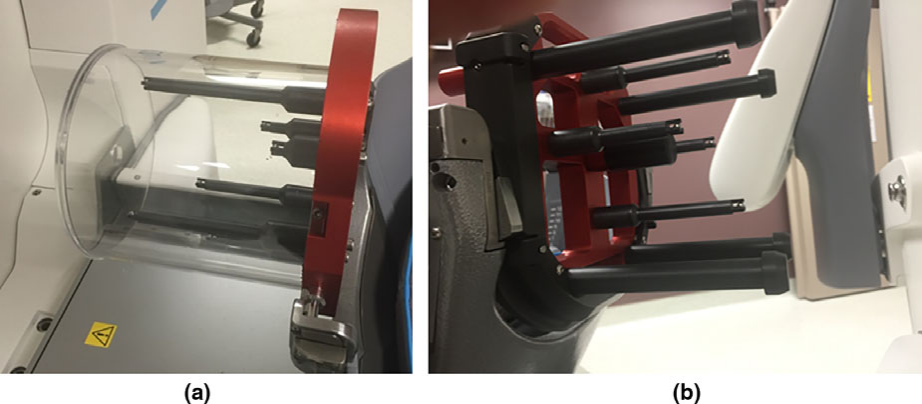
(a) The manufacturer's CBCT tool used for calibrating the CBCT position, and (b) the QA tool Plus used in daily CBCT precision tests.
